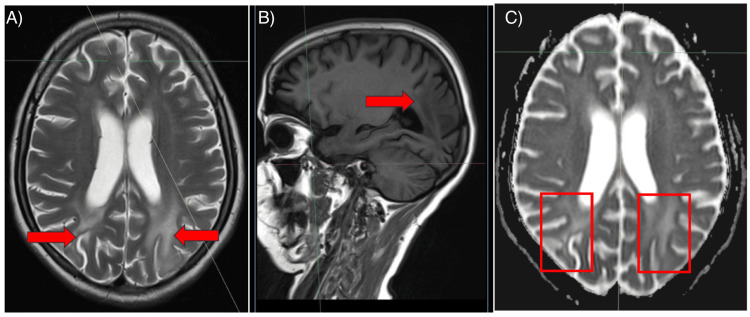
The given MRI image consists of three different sequences commonly used in neuroimaging: Image a) (T2-weighted MRI) – This image shows hyperintense (bright) areas in the parieto-occipital white matter (marked by arrows), which is characteristic of posterior reversible encephalopathy syndrome (PRES). Image b) (T1-weighted MRI) – This sagittal T1-weighted. Image c) (Diffusion-Weighted Imaging [DWI]) – This image shows restricted diffusion in the parieto-occipital regions (highlighted in red boxes). Images taken with permission and written consent of patient relatives. Image credits: Dr. Mahima Mehra, Postgraduate Resident, Department of Medicine, Maulana Azad Medical College.
